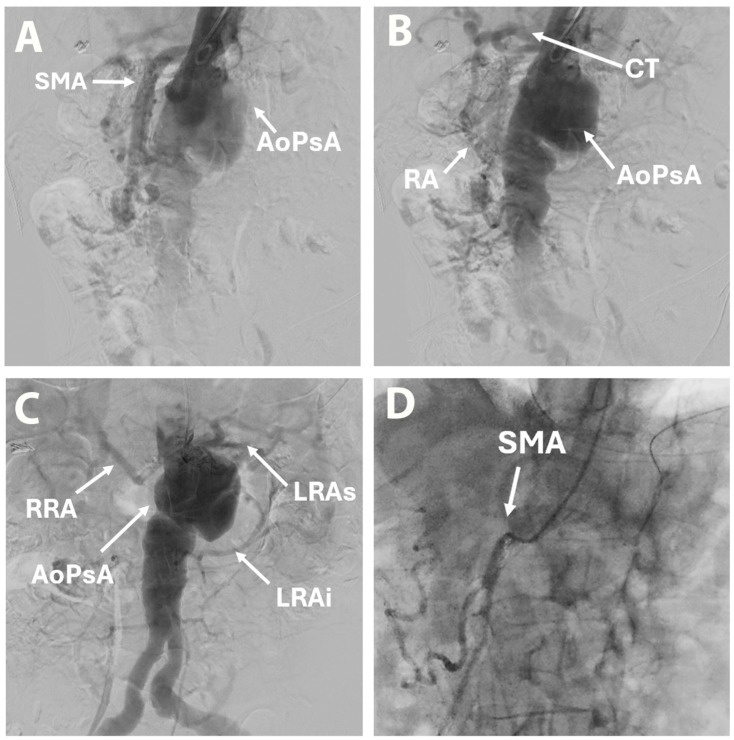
Aortography: initial view of mesenteric artery and aortic pseudoaneurysm (A), view of celiac trunk by retrograde flow from the superior mesenteric artery through the Riolan arcade (B), renal arteries distribution (C), selective arteriography of superior mesenteric artery (D). AoPsA—aortic pseudoaneurysm; CT—celiak trunck; LRAi—left renal artery inferior branch; LRAs—left renal artery superior branch; RA—Riolan arcade; RRA—right renal artery; SMA—superior mesenteric artery.
